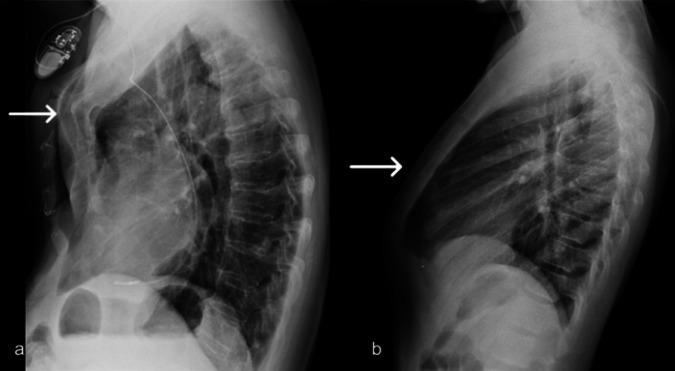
Pectus carinatum. Lateral chest X-ray views demonstrate the two types of sternal protrusion: chondromanubrial with protrusion of the manubrium and upper sternal portion (a), and chondrogladiolar with protrusion of the median and inferior region of the sternum (b)
